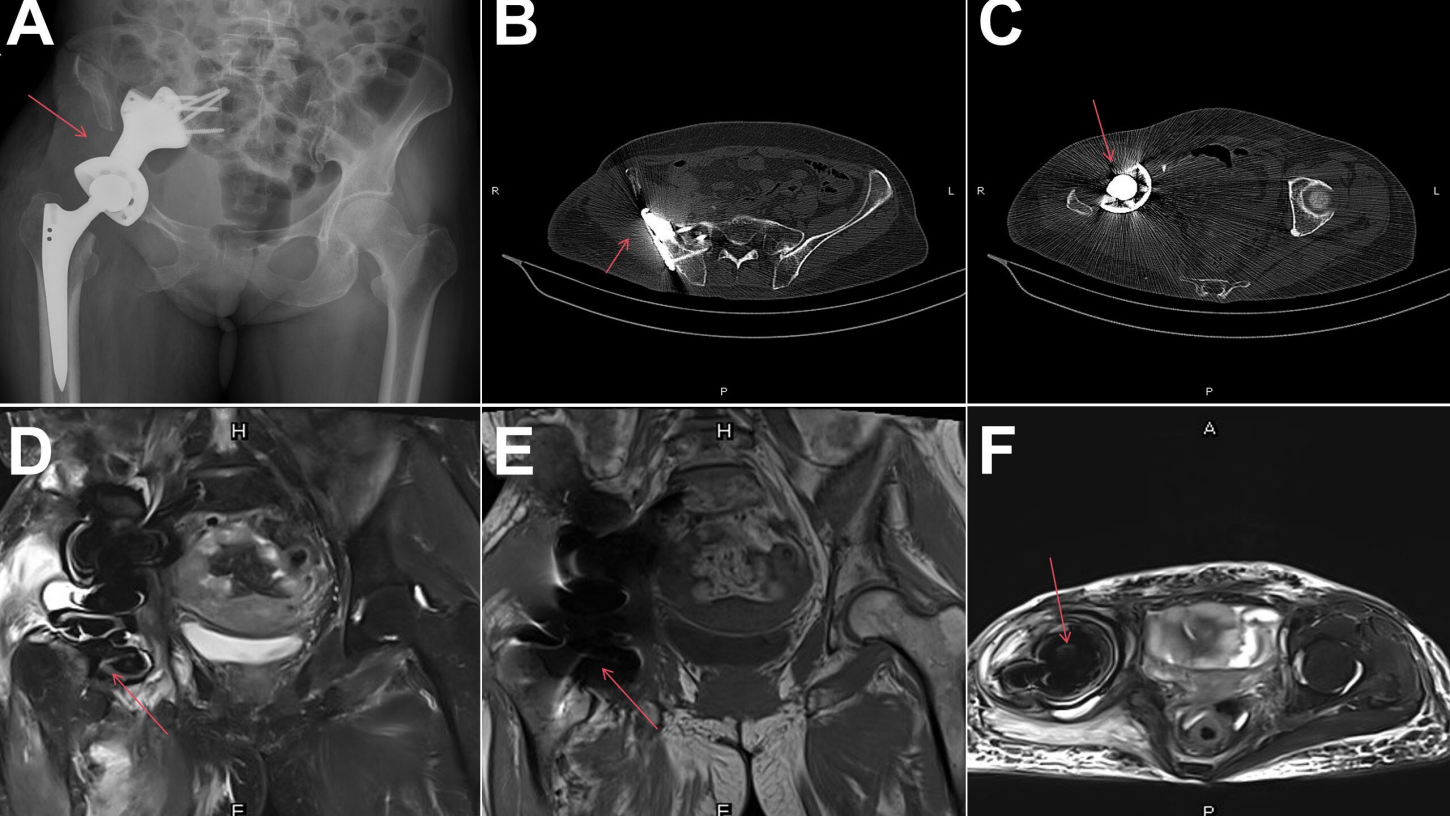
Twelve-month follow-up imaging. (A–F) Follow-up X-ray, CT, and MRI scans after 12 months: no signs of recurrence or metastasis were observed.
